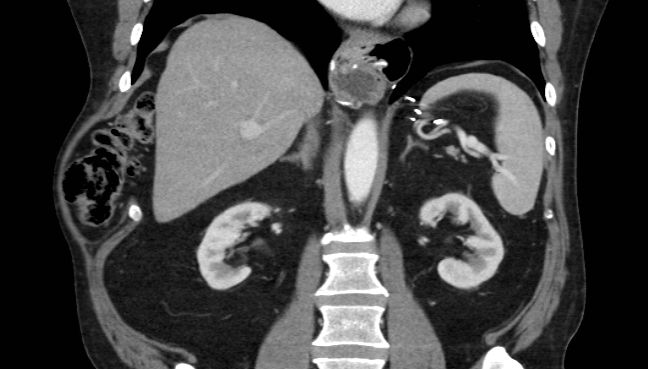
CT Scan of Patient #1 Highlighting Defect Between Anterior Ribs 9-10 and Cecum, Ascending Colon, and Omental Fat
